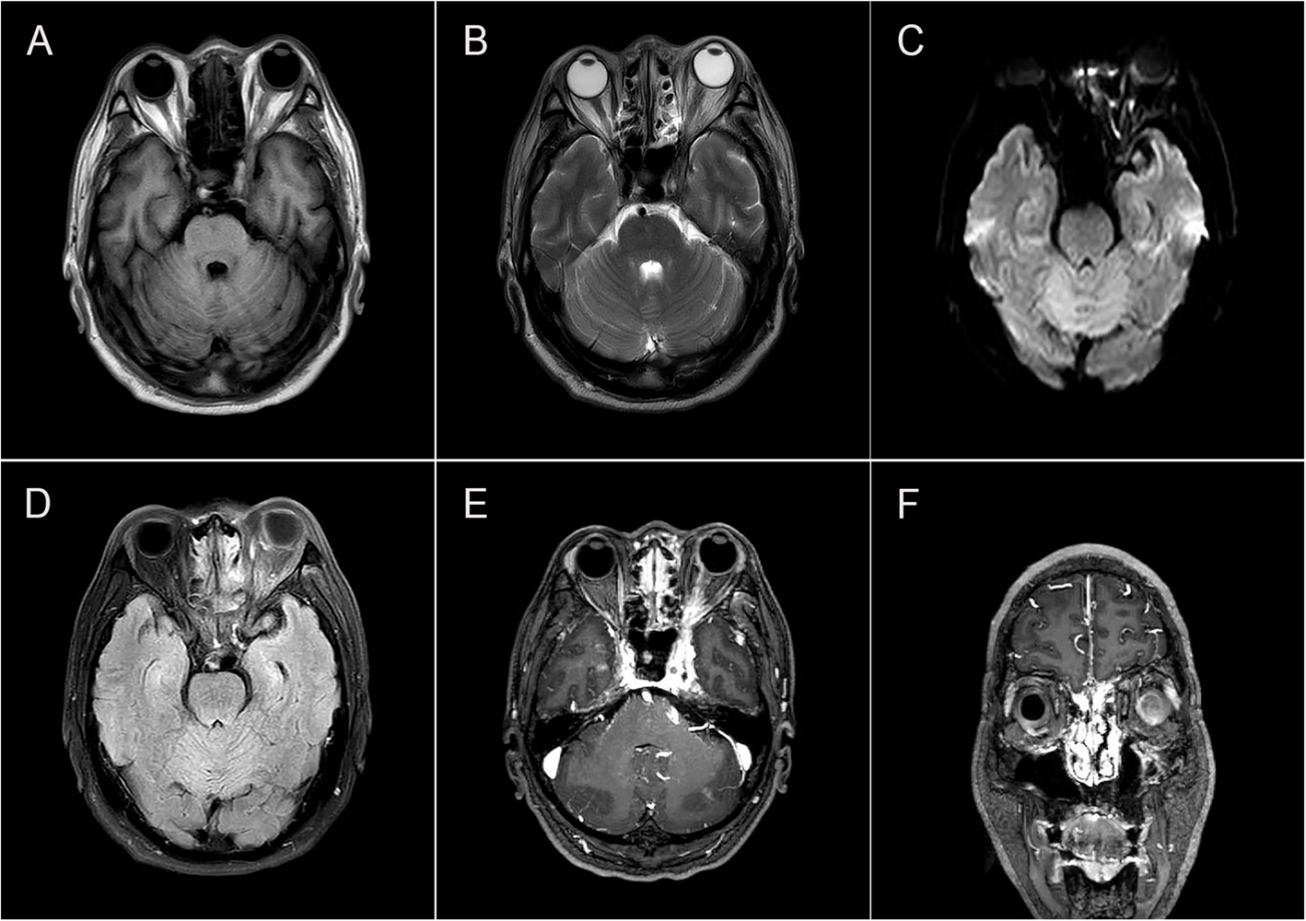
MRI of brain and orbits with gadolinium contrast showing thickened and enhanced medial, lateral, inferior, and supraocular muscles. Both T1- and T2-weighted imaging were hypointense A, B. DWI and FLAIR sequences were hyperintense C, D Axial and coronal scans were markedly enhanced E, F
